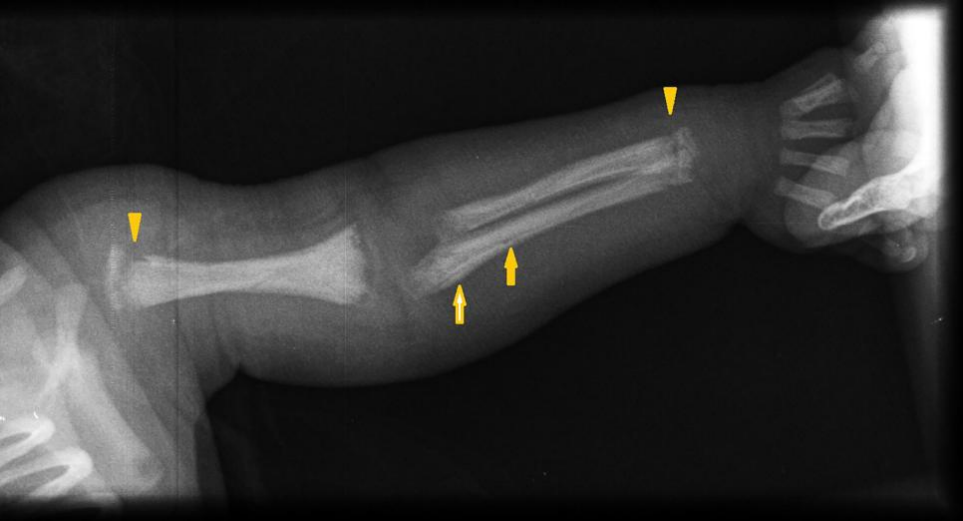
Radiographic evidence of periostitis and metaphyseal bands in upper left extremity long bones.
